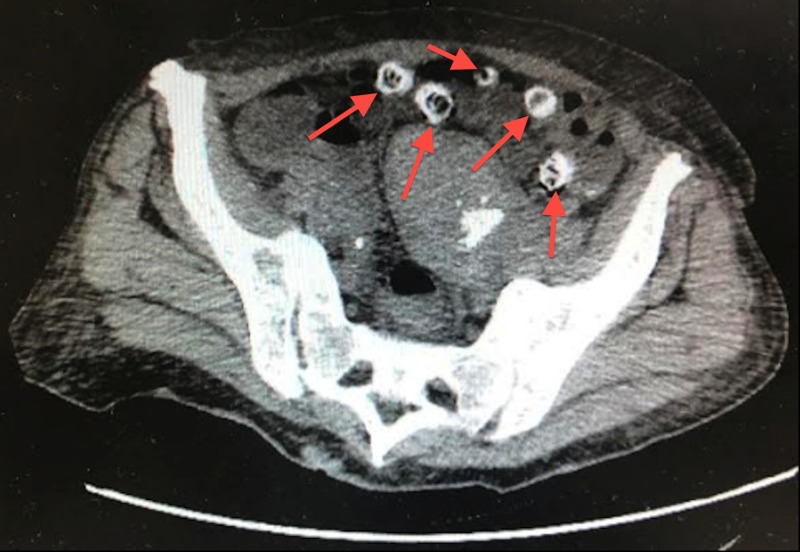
Axial computed tomography (CT) without contrast of calcified diverticula in the transverse colon causing rectal bleeding
